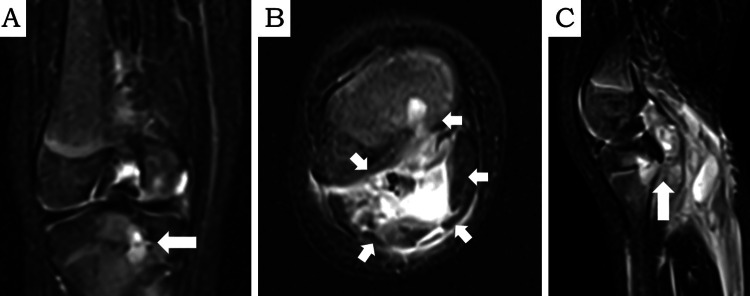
Fat-suppressed gadolinium-enhanced T2-weighted magnetic resonance imaging (MRI) obtained at admission A: Coronal view of the right tibia showing a high-intensity lesion in the bone marrow of the proximal tibial metaphysis and epiphysis (arrow). B: An axial MRI shows a subcutaneous abscess affected by peripheral enhancement by gadolinium. Abscess formation extending from the osteomyelitis lesion to the intermuscular and subcutaneous areas was observed (arrow). C: Sagittal MRI shows that the subcutaneous abscess is continuous with the osteomyelitis lesion (arrow).
